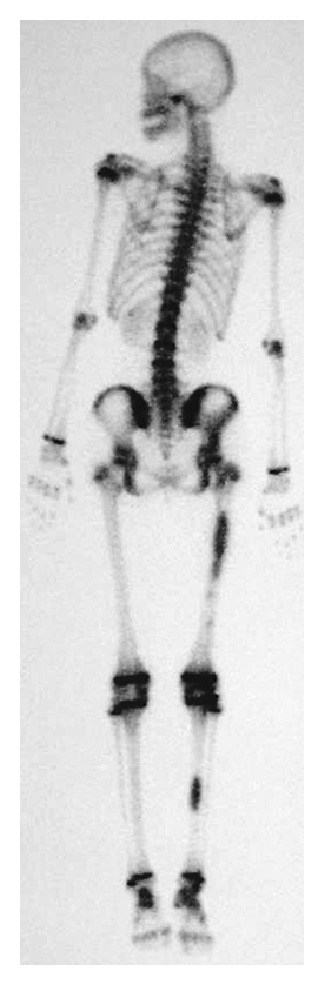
Technetium bone scintigram. Abnormal uptakes are shown in the diaphyses of the right femur and the right tibia and around the bilateral knees and ankles.
